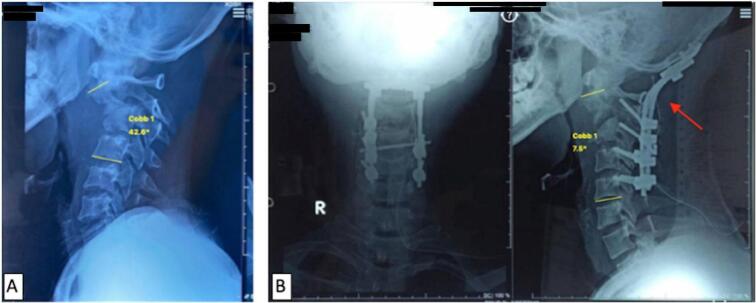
(A) Pre-operative lateral cervical X-ray; (B) Immediate postoperative anteroposterior and lateral cervical X-ray using posterior stabilization with occipital-cervical fusion (red arrow). (For interpretation of the references to color in this figure legend, the reader is referred to the web version of this article.)
